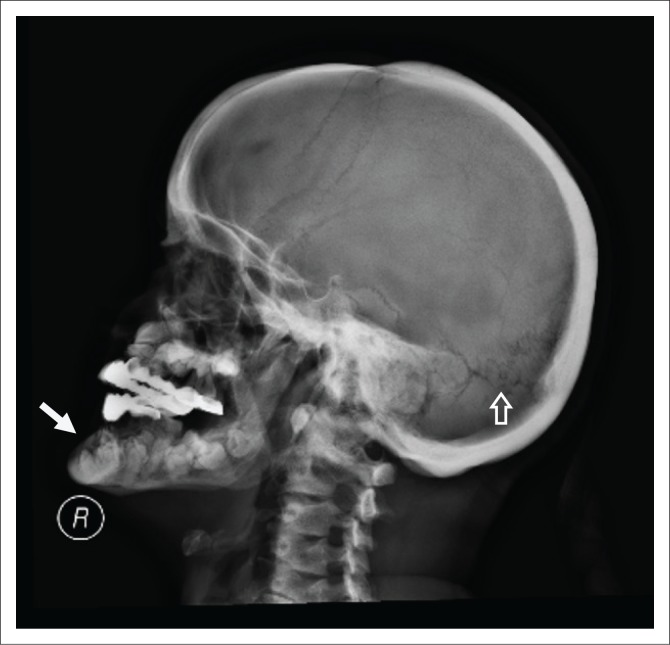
Lateral radiograph of the skull shows normal bone density with wormian bones in the lambdoid suture (hollow arrow), prognathism and supernumerary teeth (white arrow). Patient is wearing dental prosthesis.
